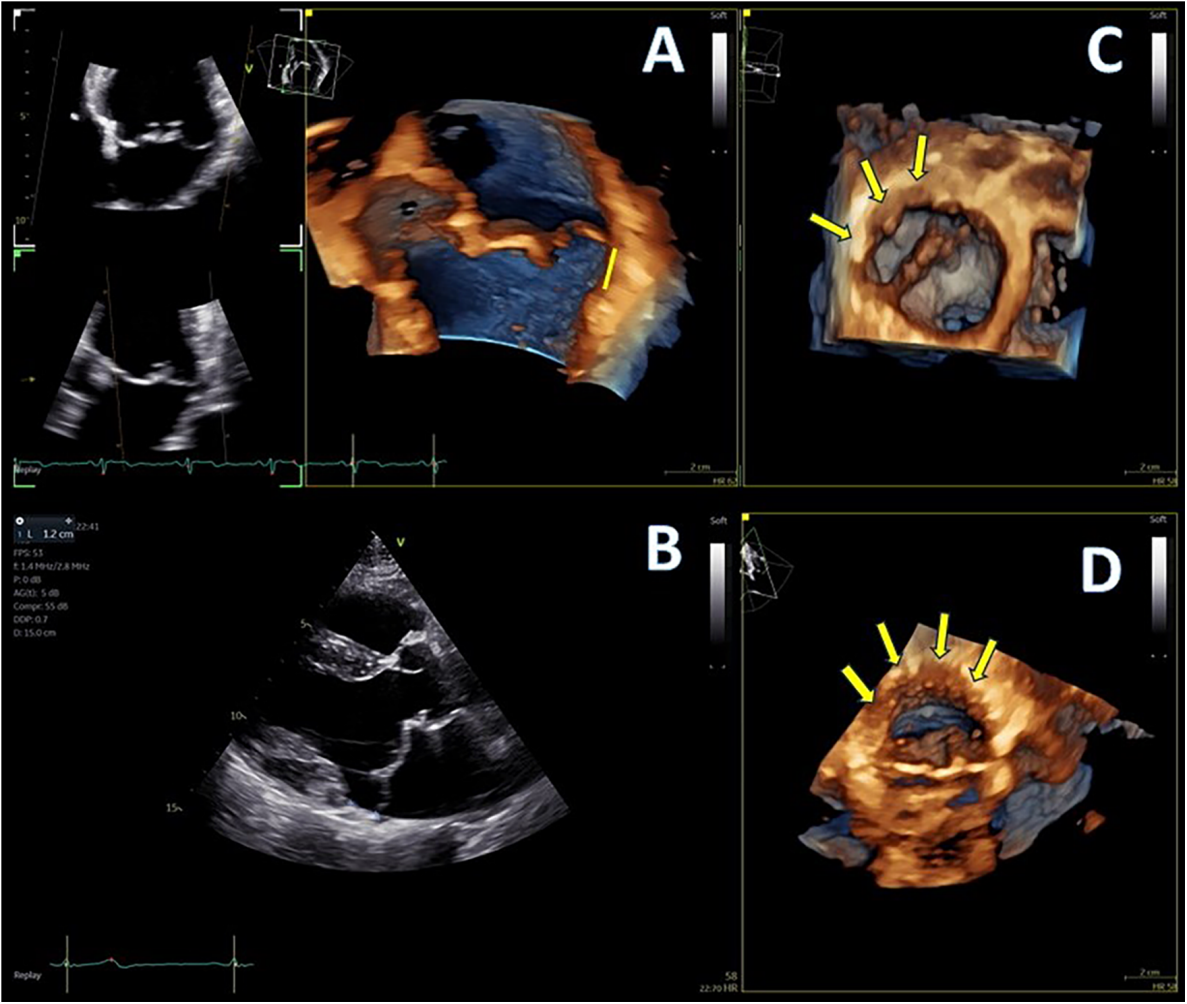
(A,B) Mitral annular disjunction (MAD) demonstrated by 4D and 2D transthoracic echocardiography (TTE), 12 mm of distance from posterior left ventricular (LV) wall, indicated with yellow line, and (C,D) extent of disjunction arc seen with two different orthogonal volume rendering views, indicated with yellow arrows.
